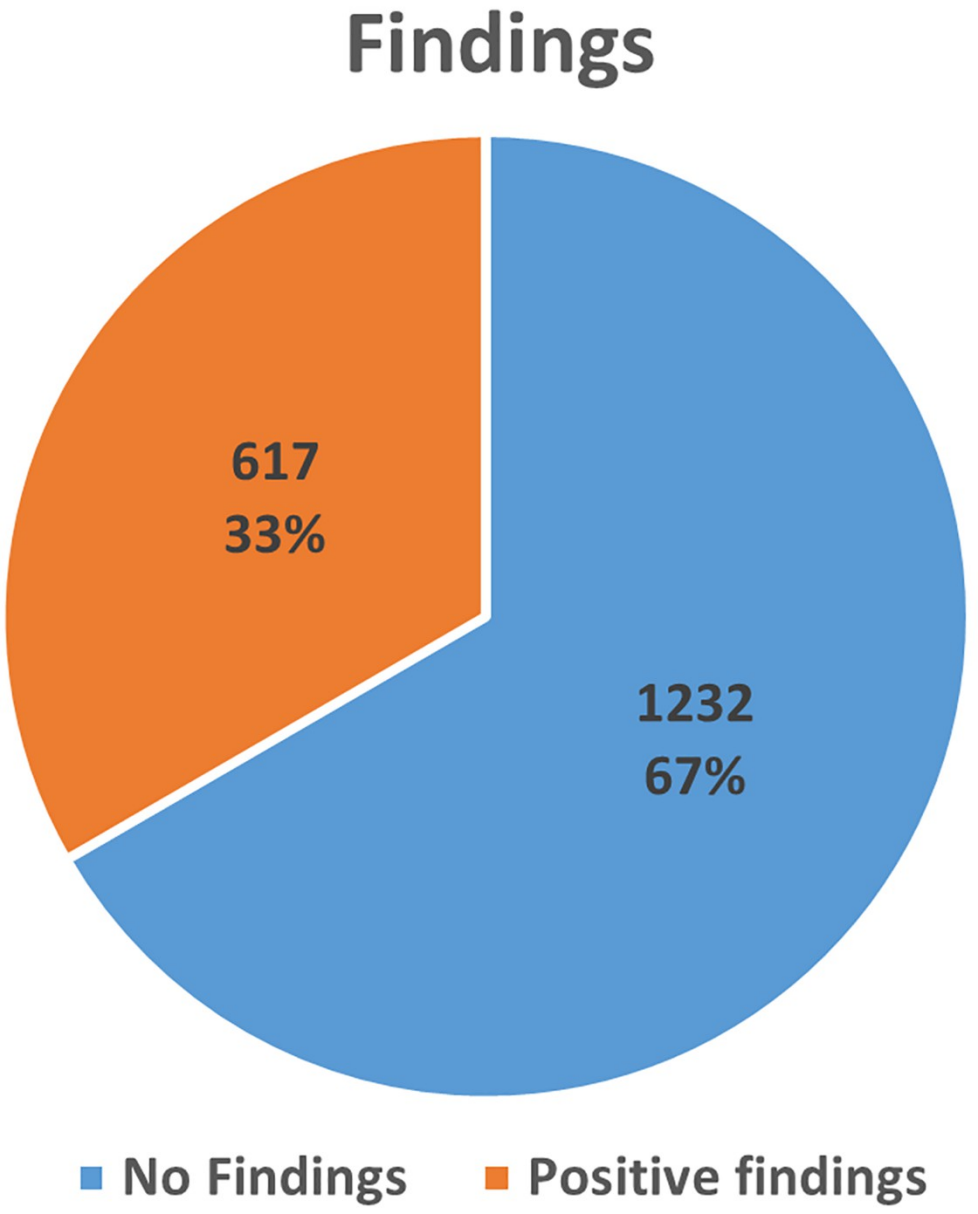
Prevalence of the paranasal sinuses among the patients. After assessing the CT scans, the data showed that the most frequently affected were maxillary sinuses, with a total (31.7%, n = 586) of all positive cases. unilateral maxillary antrum sinusitis was the most frequent pathologies (20.49%, n = 249) of all positive findings, maxillary polyps (Fig 3).
